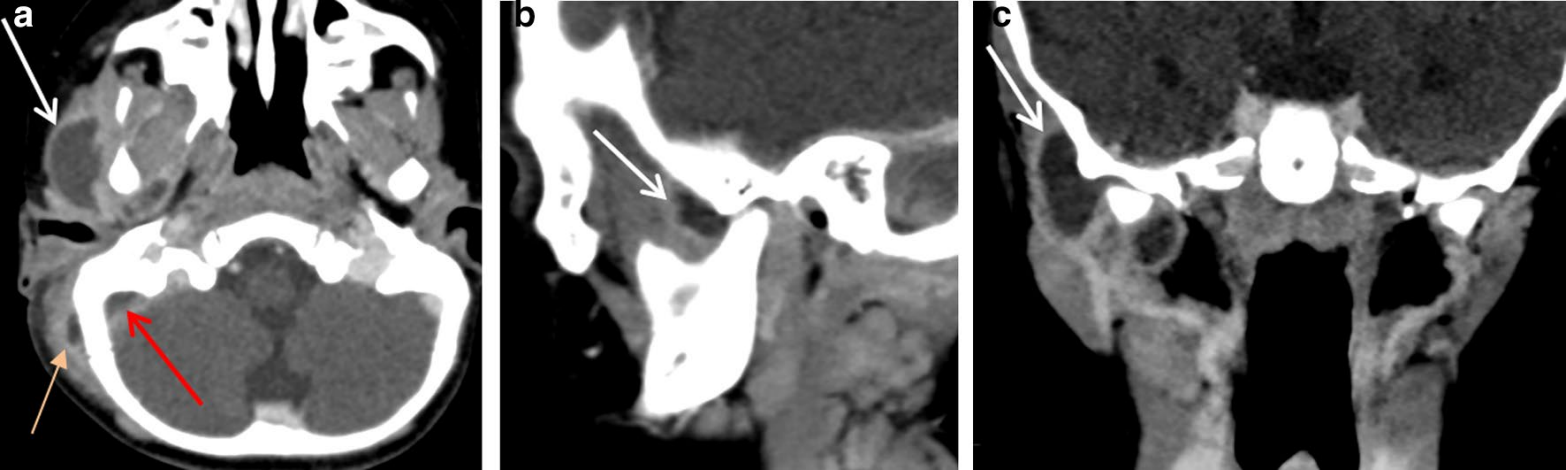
Fusobacterium necrophorum infection. Right otomastoiditis complicated by subperiosteal abscess [orange arrow], sigmoid sinus thrombosis [red arrow] and temporomandibular arthritis [white arrow] leading to cheek swelling, on axial, parasagittal and frontal CT images, after intravenous injection of contrast medium (a, b and c)
